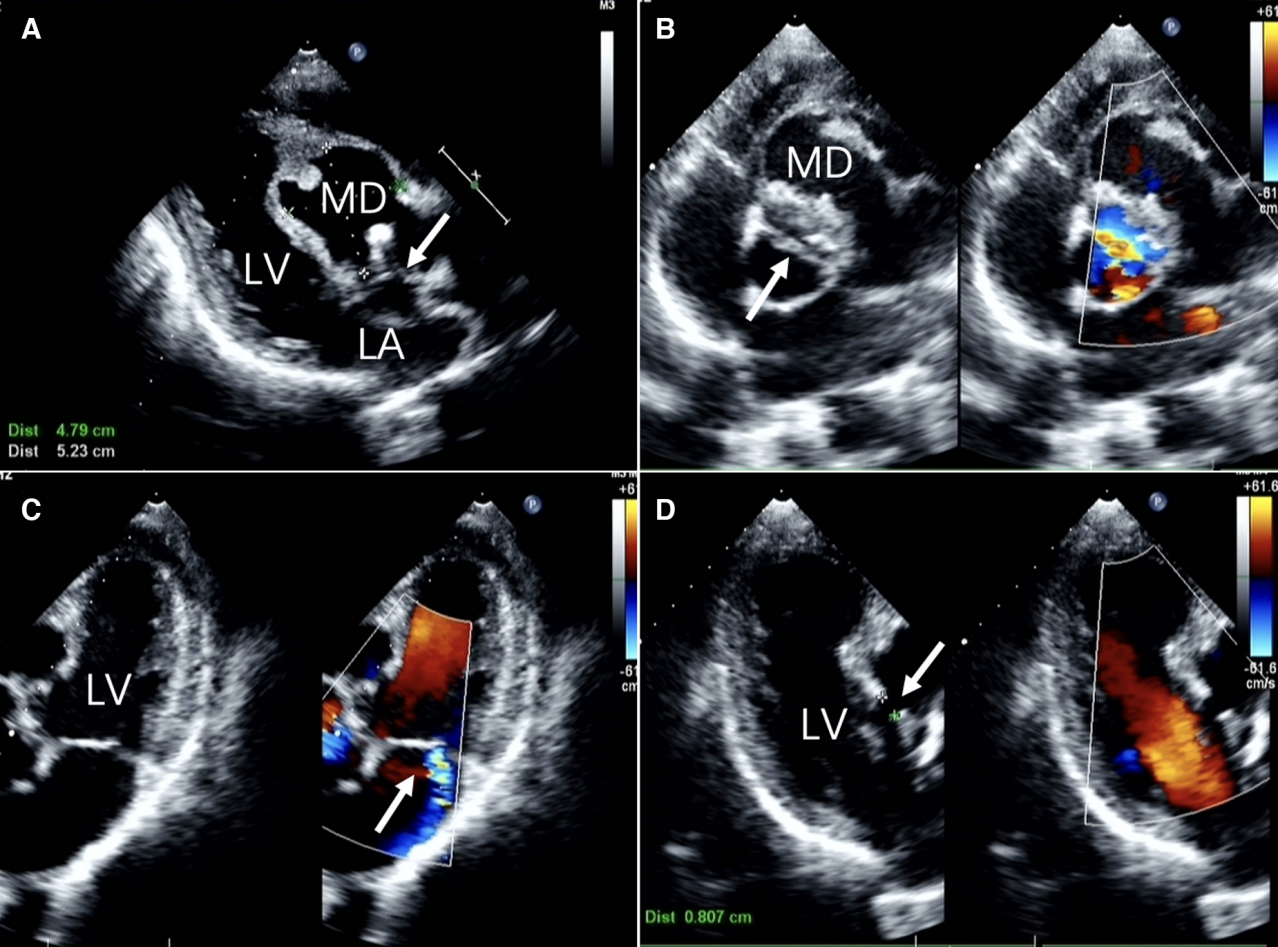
Two-dimensional transthoracic echocardiographic images (A) long-axis view of the left ventricle showing echo interruption, no echo in the interventricular septum.(white arrow) (B) the short-axis cross-sectional view of the aorta demonstrates a bicuspid aortic valve anomaly. (white arrow) (C) the non-standard pentachamber cardiac section reveals mitral valve eccentric regurgitation. (D) The three-chamber cardiac cross-section reveals the dimension of the orifice. LA, left atrium; LV, left ventricle; MD, Myocardial dissection.
